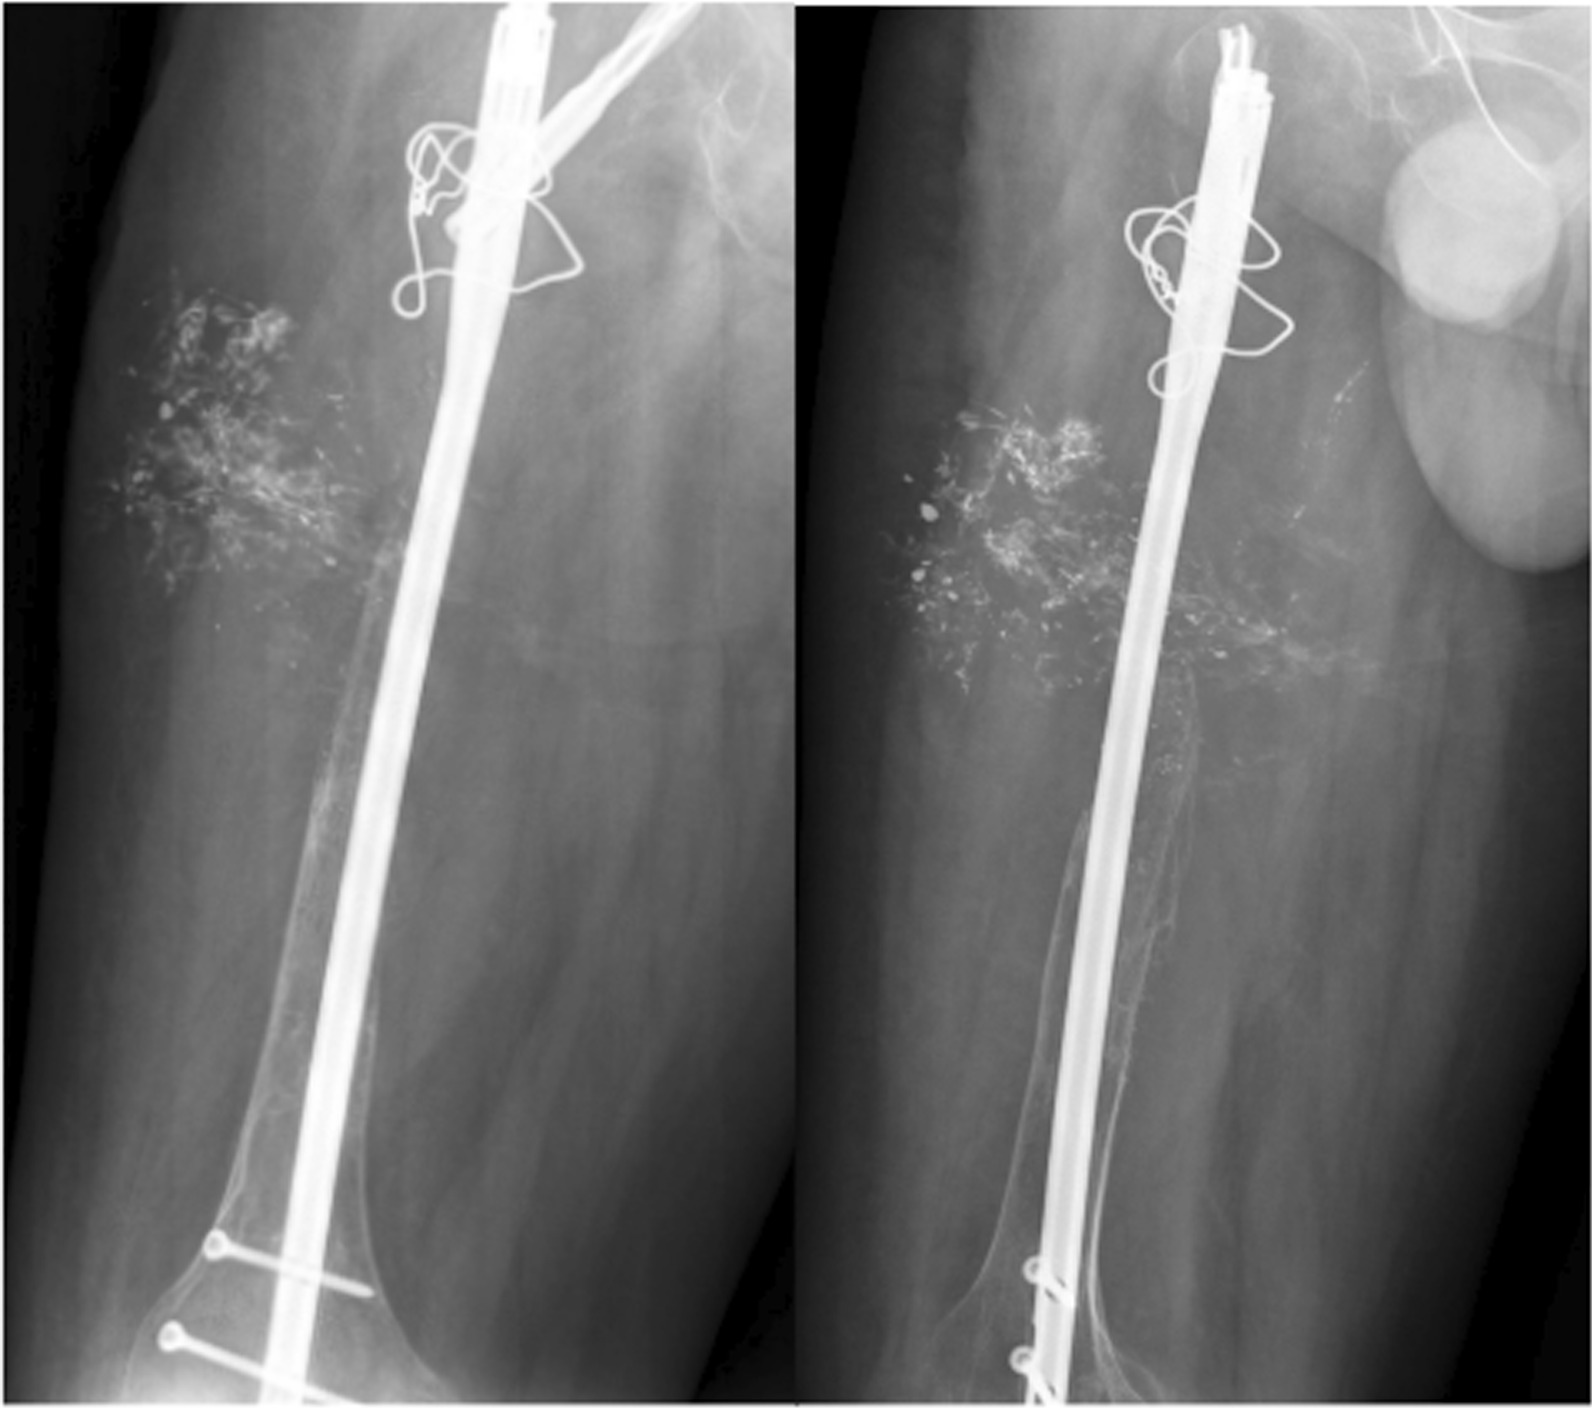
Right femoral X-ray of patient when he was referred to us
